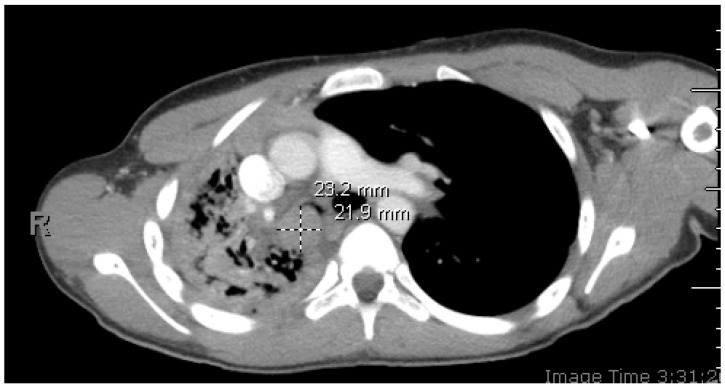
Initial chest CT with contrast: axial image depicting right mainstem bronchus intraluminal mass. A 14-year-old healthy patient presented with cough, congestion, abdominal pain, and chest tightness worsening over several weeks. She denied any contact with people with similar symptoms or recent travels and received all recommended vaccinations. She reported two episodes of hemoptysis about 1.5 years prior. The first episode was associated with cough and wheezing for one day. The second episode was associated with similar symptoms, and she was given antibiotics to treat for infection at that time. Upon examination, the patient was tachycardic with a heart rate of 130 beats/min, tachypneic with a respiratory rate of 30 breaths/min, and hypoxic with an oxygen saturation of 74% on room air, therefore requiring a non-rebreather mask for oxygenation. Lung examination revealed coarse, significantly diminished breath sounds throughout the right side. CT imaging revealed a 2.3 × 1.9 × 3.0 cm soft tissue lesion obstructing the right mainstem bronchus with resultant atelectasis of the right lower lobe, right-sided tracheal deviation with mediastinal shift, and patchy airspace disease throughout the right middle and upper lobes concerning for post-obstructive pneumonia.
